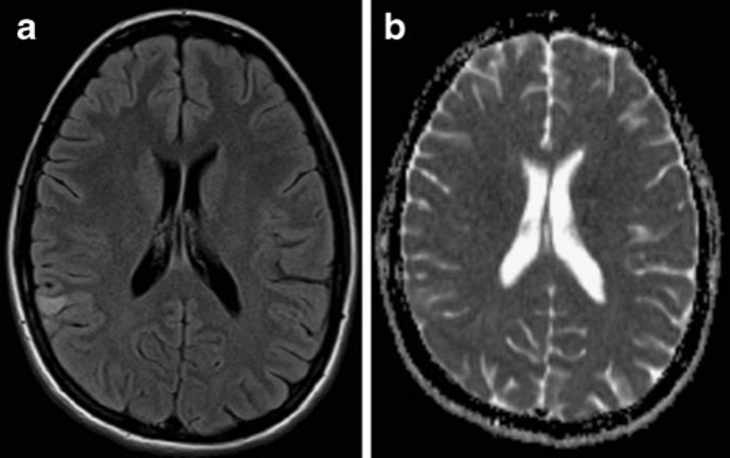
A 20-year-old female with sudden onset of neurological symptomatology. a FLAIR image shows an area of increased signal intensity in the right angular region. b ADC map does not show restricted diffusion that would be characteristic for an acute ischemic stroke. Encephalitis was proven by CSF examination. FLAIR, fluid attenuated inversion recovery; ADC, apparent diffusion coefficient; CSF, cerebrospinal fluid
